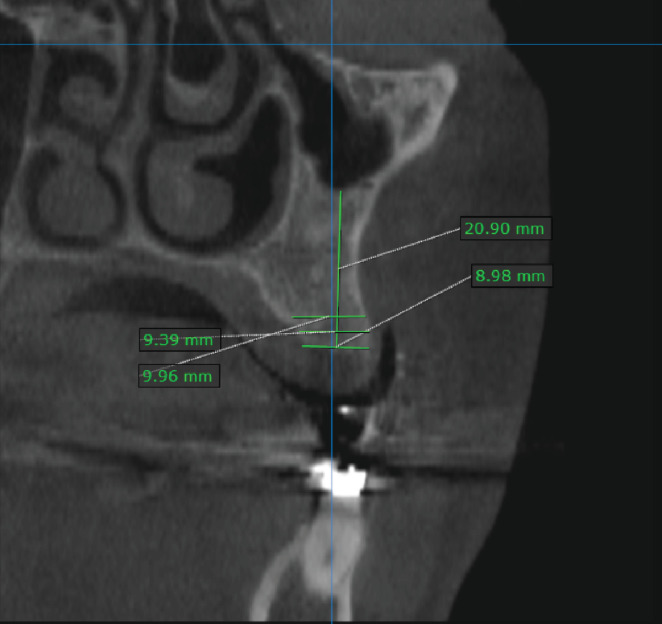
The radiographic measurements after 6 months of the surgical procedures.
